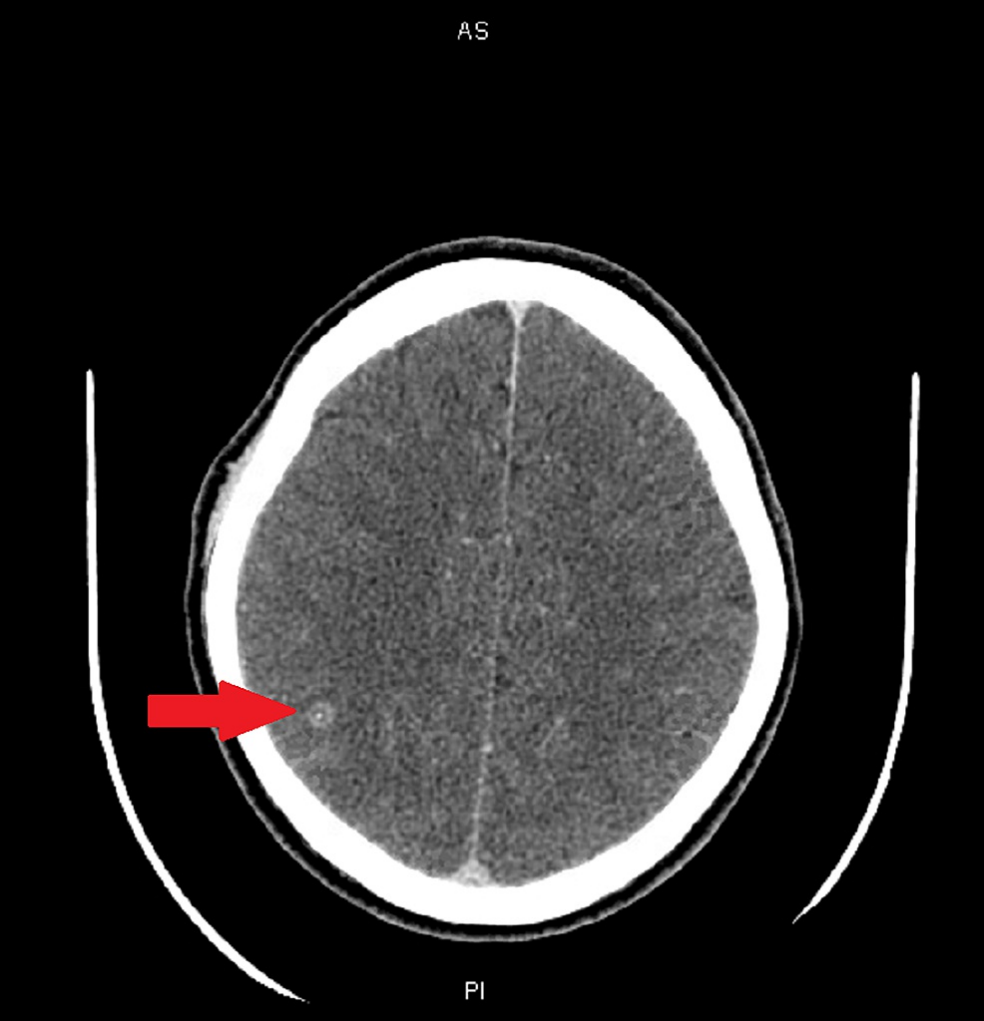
CT of the head without contrast showing a 7-mm ring-enhancing lesion with a dot-like intrinsic calcification along the right parietal lobe.
